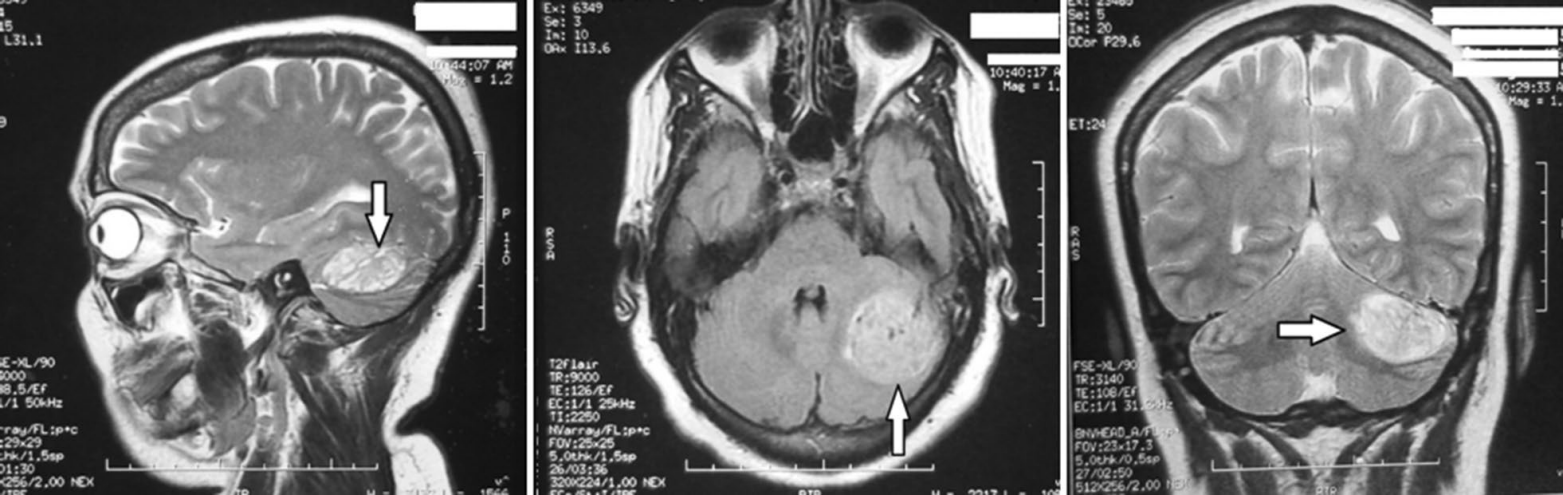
Three MRI images showing an expansive intra-axial mass of the *left* cerebellar lobe, with no mass effect on the ventricular system, measuring 37 × 35 × 23 mm
